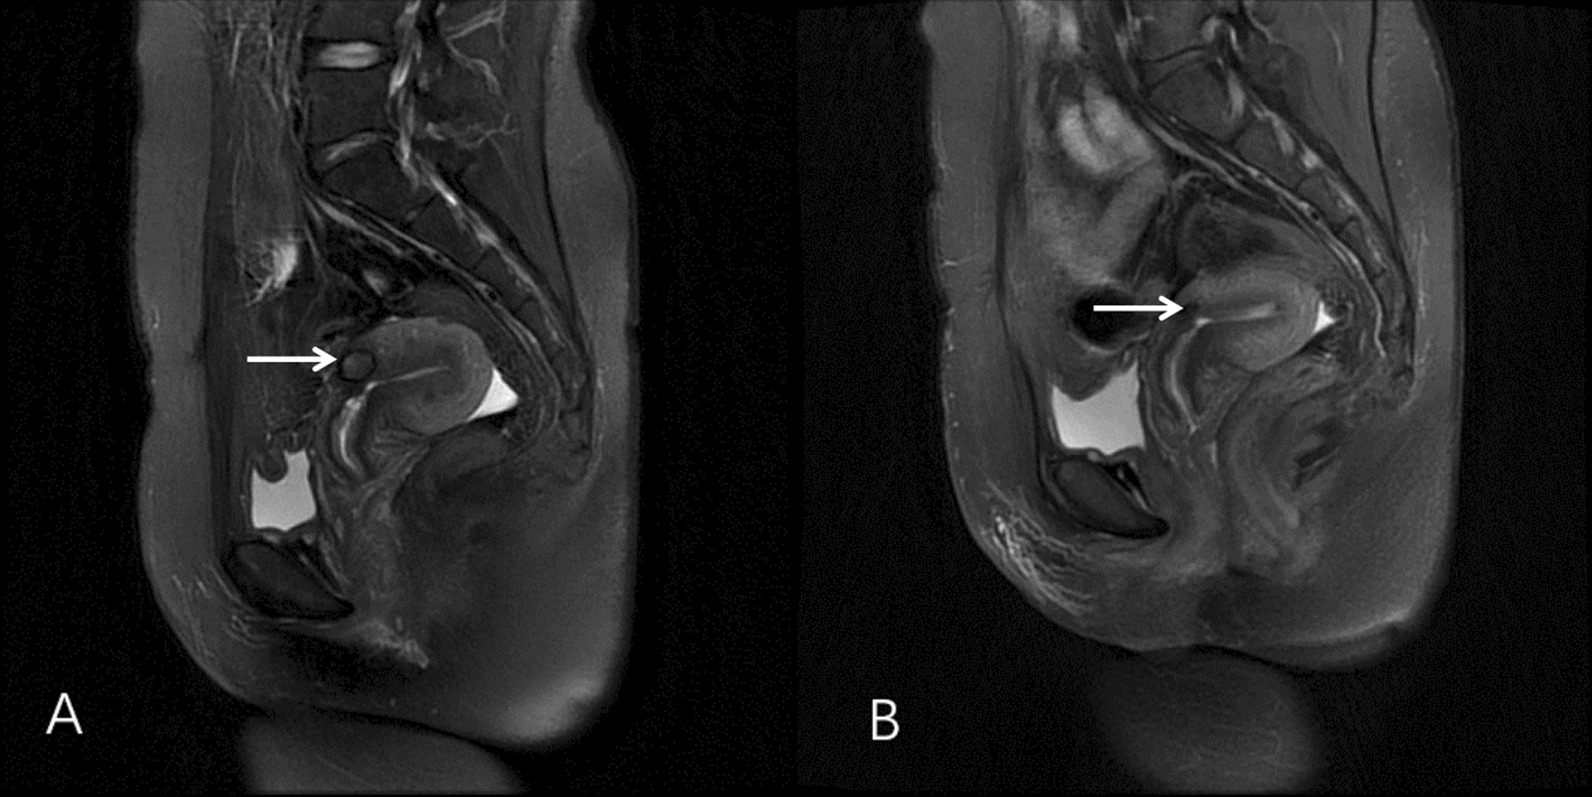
MRI of the uterine lesion pre-HIFU (A) and post-HIFU (B) treatment. Sagittal T2 image showing the uterine lesion of GTN located in the cesarean scar (A). Only cesarean scar defect was seen at 4 months after HIFU treatment (B). MRI, magnetic resonance imaging; HIFU, high-intensity focused ultrasound; GTN, gestational trophoblastic neoplasia
